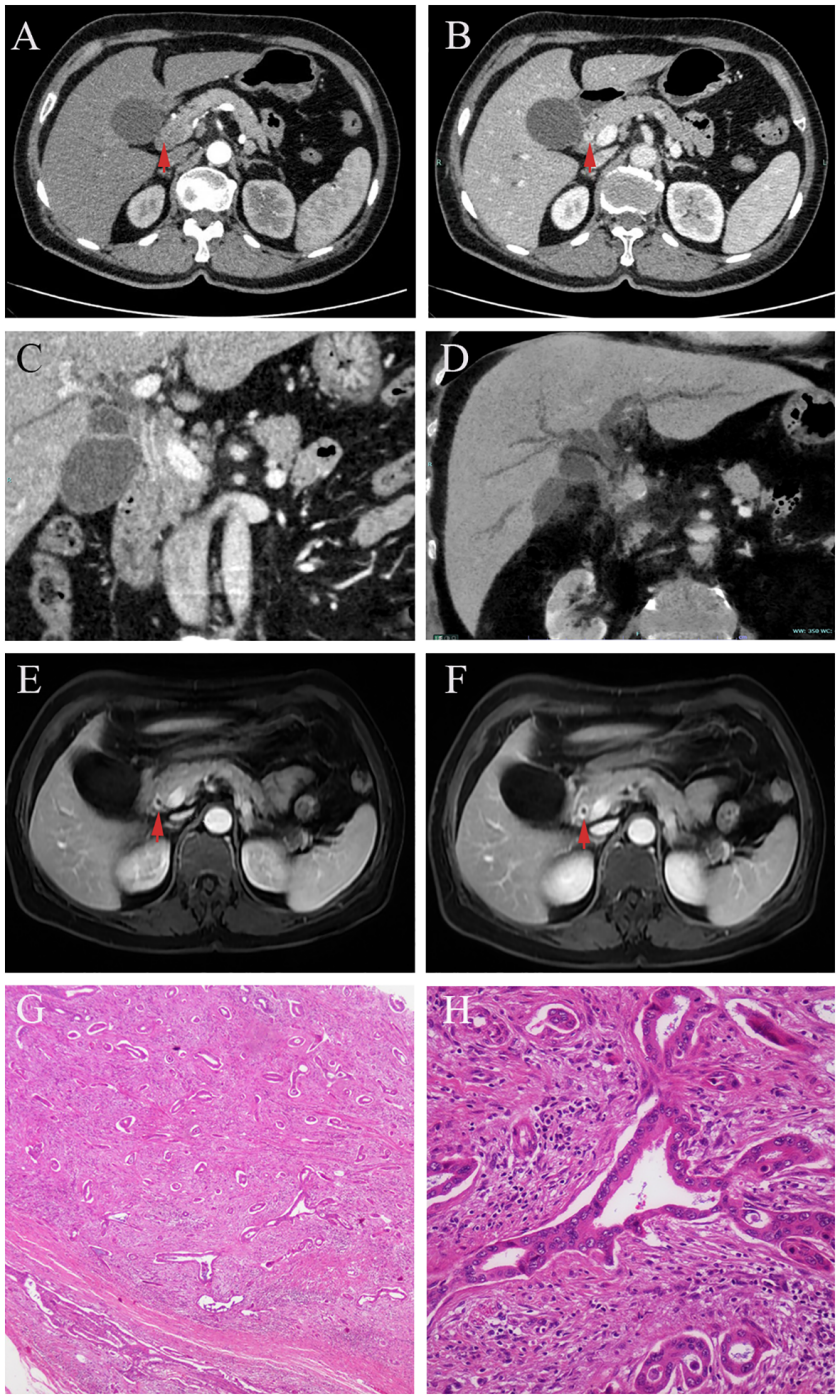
In DCE-CT, the biliary wall is not obviously enhanced in arterial phase (A, red arrow), but circular enhancement is seen in portal phase (B, red arrow). Multiplanar reconstruction (MPR) in the portal phase of DCE-CT, the wall of the common bile duct is thickened and strengthened at long segments. An abrupt termination of the bile duct with a distal tapering, referred to as a “choledoch cutoff sign” (C). Minimum intensity projection (MinIP) shows the vine-like expansion of the intrahepatic bile duct (D). In DCE-MRI, from the portal phase (E, red arrow)to the delayed phase (F, red arrow), the enhancement of the bile duct wall becomes evident gradually. Postoperative pathology revealed distal biliary stricture due to cholangiocarcinoma (G, H).
